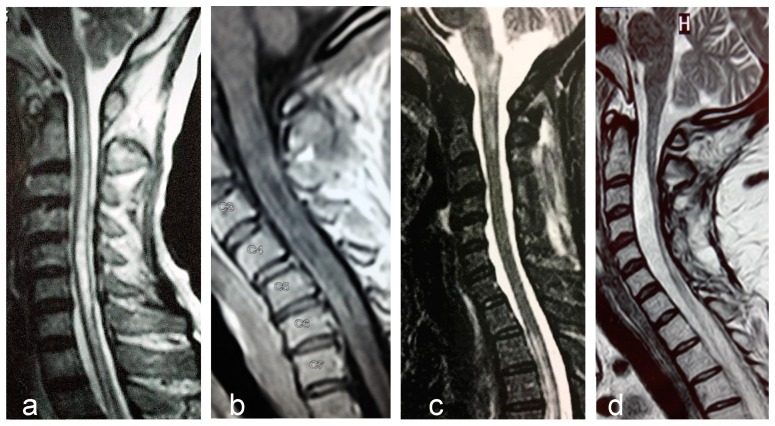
Examples of longitudinally extensive spinal cord lesions detected by MRI in AQP4- seropositive NMOSD patients. (a). T2-weighted central longitudinally extensive cervical lesion. (b). T1-weighted lesion with gadolinium showing multiple hypointensities (cavitations) throughout the cervical cord. (c). T2-weighted cervical lesion extending to brainstem. Another lesion is seen in the upper thoracic levels. (d). Longitudinally extensive spinal cord atrophy of the cervical cord.
